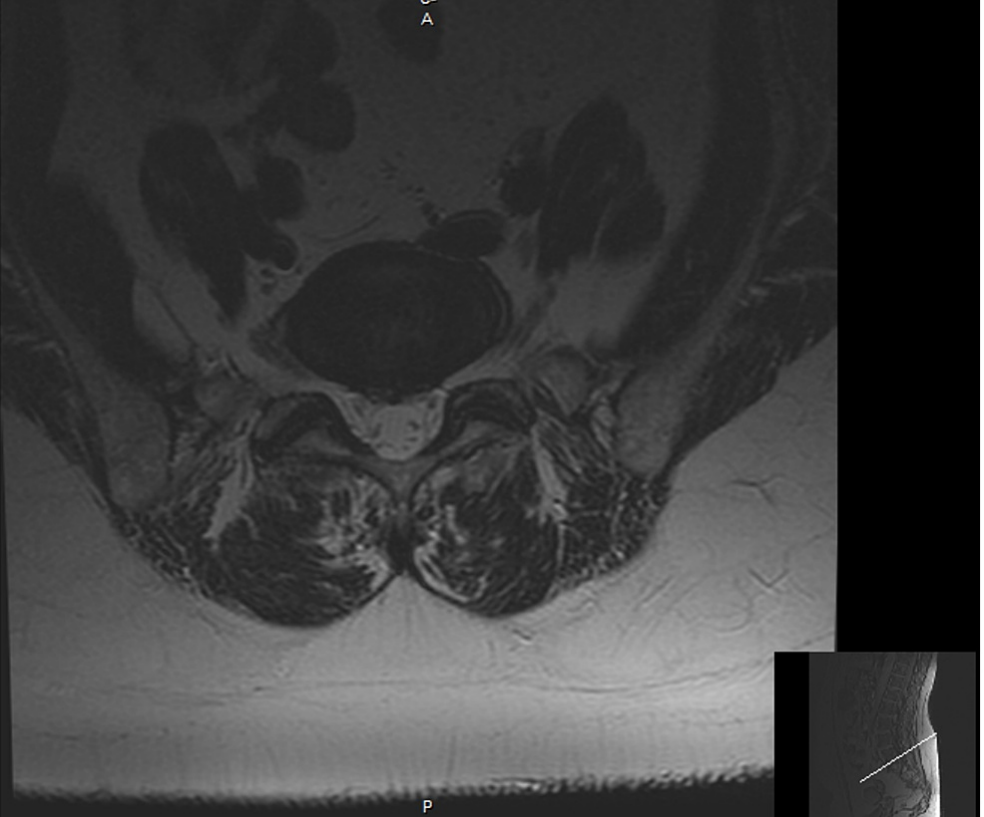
Lumbar MRI without contrast: axial view. MRI: magnetic resonance imaging
